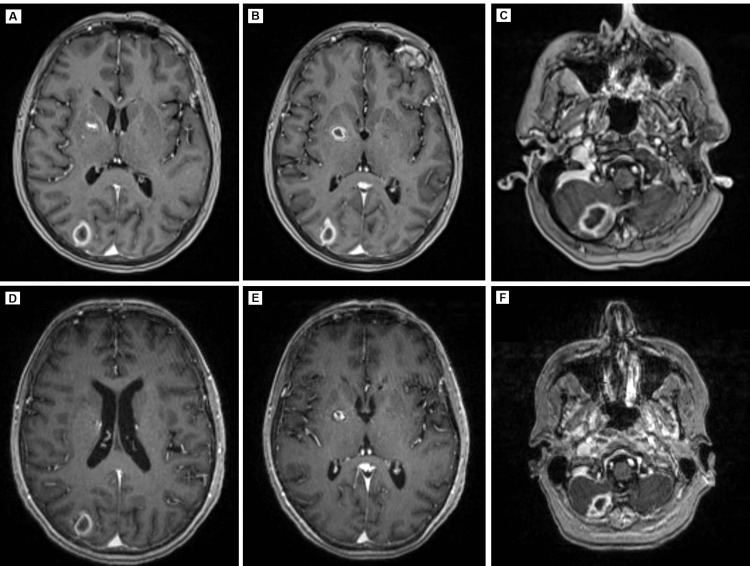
(A-C): MRI of the brain on day 12 of admission showing a 1.1 × 1.1 × 1.0 cm lesion in the right lentiform nucleus, a 1.9 × 1.3 × 1.7 cm lesion in the right occipital lobe, and a 2.5 × 1.4 × 1.6 cm lesion in the right cerebellum. (D-F): MRI of the brain on day 14 of admission showing a slight decrease in the size and no new lesions: a 9 × 7 mm lesion in the right lentiform nucleus, a 1.4 × 1.2 cm lesion in the right occipital lobe, and a 2.2 × 1.2 cm lesion in the right cerebellum. MRI: magnetic resonance imaging
